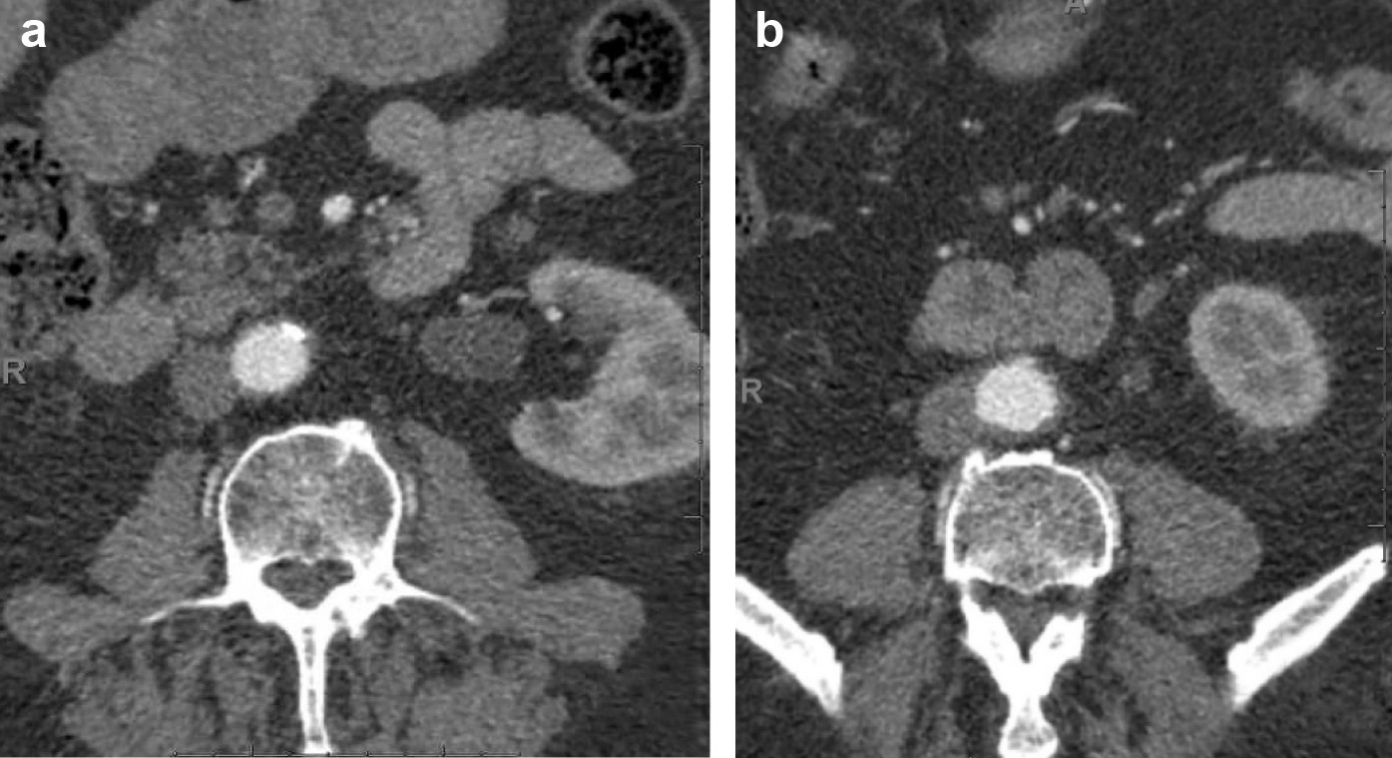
a,b, Computed tomography (CT) scans at 12 months demonstrating resolution of retroperitoneal soft tissue changes following treatment with corticosteroids and mycophenolate mofetil.
